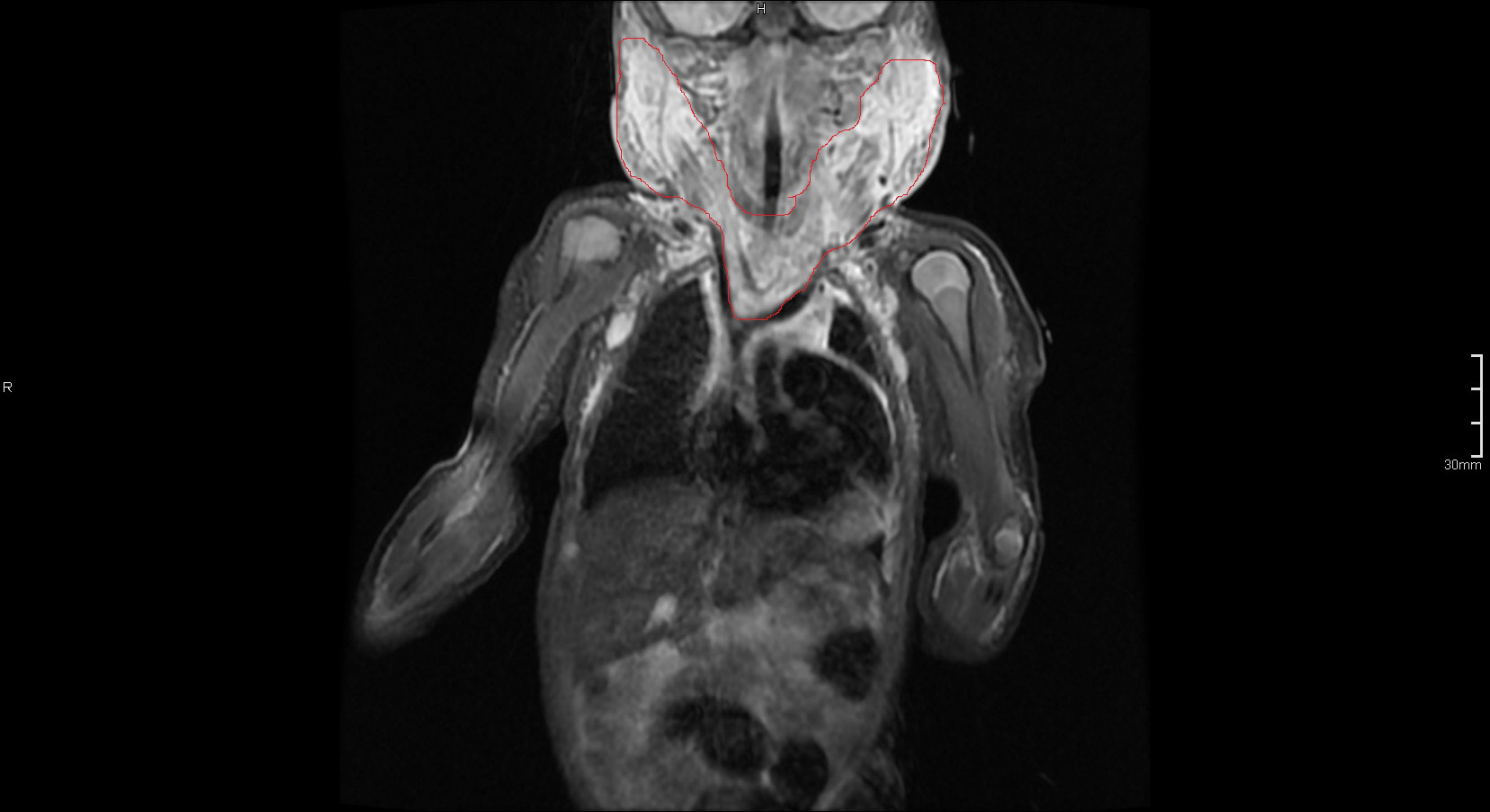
Magnetic resonance imaging: Short T1 inversion recovery (STIR) sequence where the tumor edema is depicted in white.
